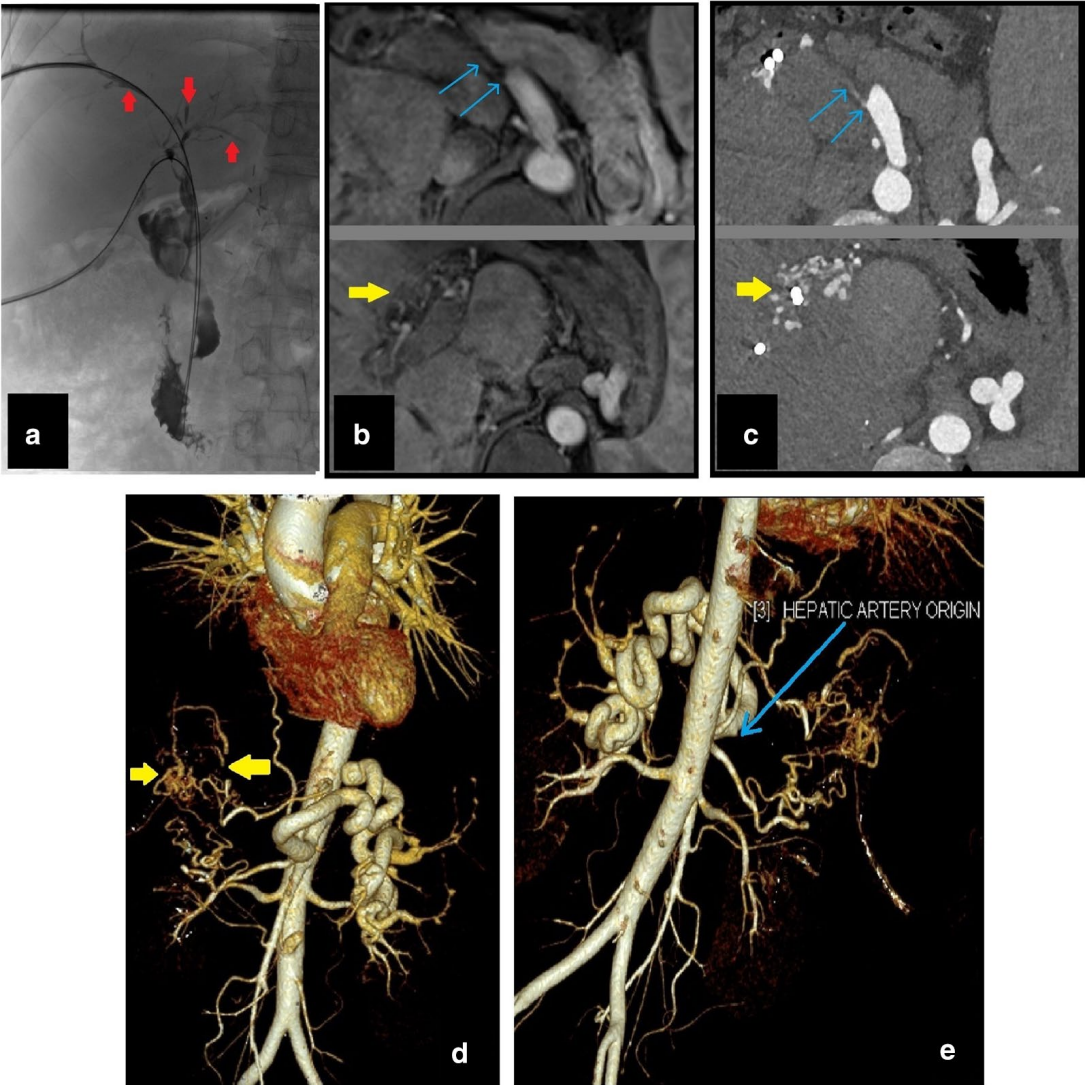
Delay in diagnosis with no apparent harm. A 45-year-old male patient presented at the clinic with jaundice and recurrent cholangitis history after liver transplantation. Due to persistent itching and progressive jaundice, percutaneous transhepatic cholangiography (PTC) was performed for biliary drainage. PTC showed multisegmental involvement with dilated and stenotic biliary ducts (red arrows, a). Contrast-enhanced upper abdominal MRI and MRCP for evaluation of the biliary tree were reported as “Chronic cholangiopathic changes due to recurrent episodes of cholangitis” without any differential diagnoses. The clinicians suspected "ischemic cholangiopathy" due to liver transplant history and ordered abdominal CTA to rule out ischemic etiology. The CTA, which was performed two weeks after MRI, revealed hepatic artery occlusion (blue arrows, c, e) and arterial collateralization (yellow arrows, c, d). After confirmation of “ischemic biliopathy”, the previous MRI was evaluated retrospectively. The MRI images (b) taken from the same slice levels of CTA (c) were less obvious to diagnose due to artifacts secondary to inadequate breath-holding. Higher spatial resolution with shorter imaging time of CTA enabled the radiologist to make an accurate diagnosis. As in this case, choosing the wrong technique or modality may decrease the possibility of detecting abnormal findings, cause a delay in diagnosis, and sometimes even make diagnosis impossible. However, in this particular case, the change in the diagnosis did not change the patient management to a great extent and did not cause any apparent harm
